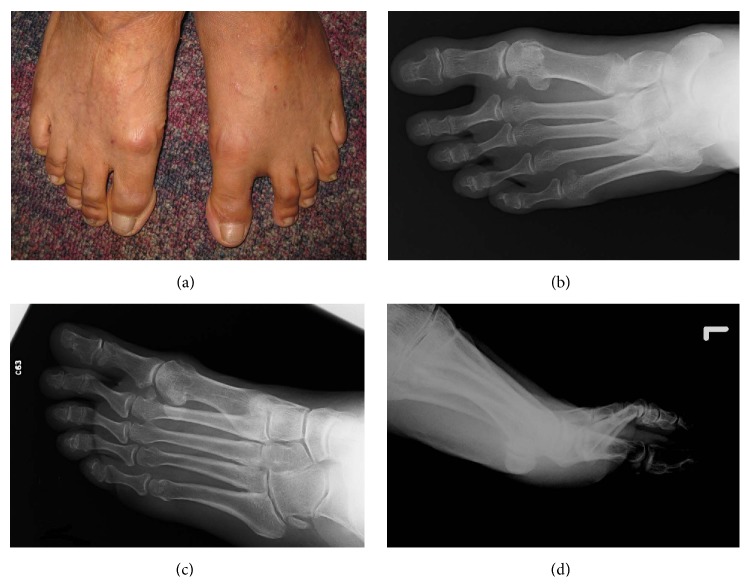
Clinical photos of this patient showed left hallux varus deformity (a). Radiographs showed avulsion fracture at the lateral side of base of proximal phalanx (b, c, and d).
